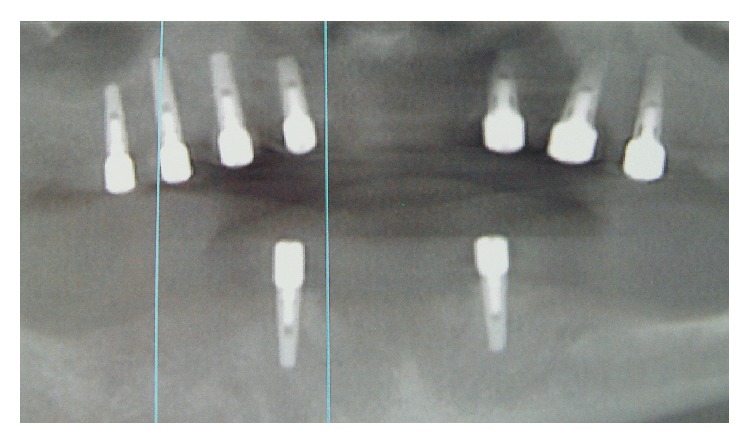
Panoramic radiograph after implant insertion.
